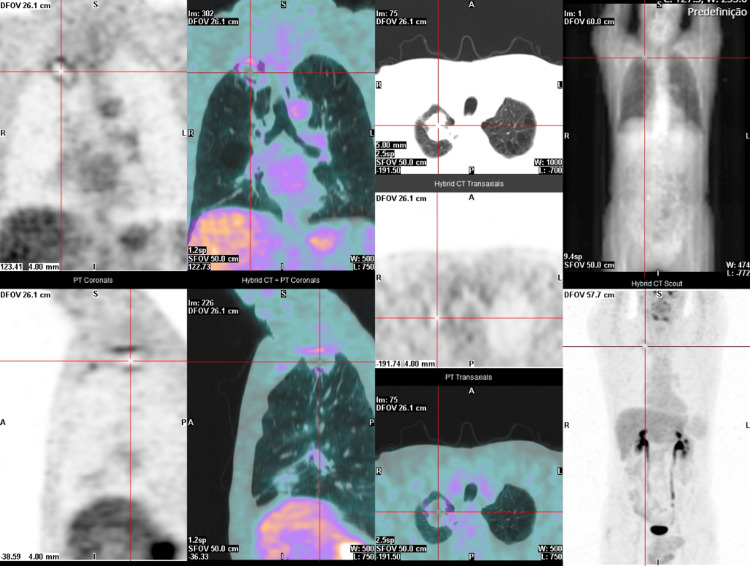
FDG/PET-CT after 16 cycles of pembrolizumab in August 2021
